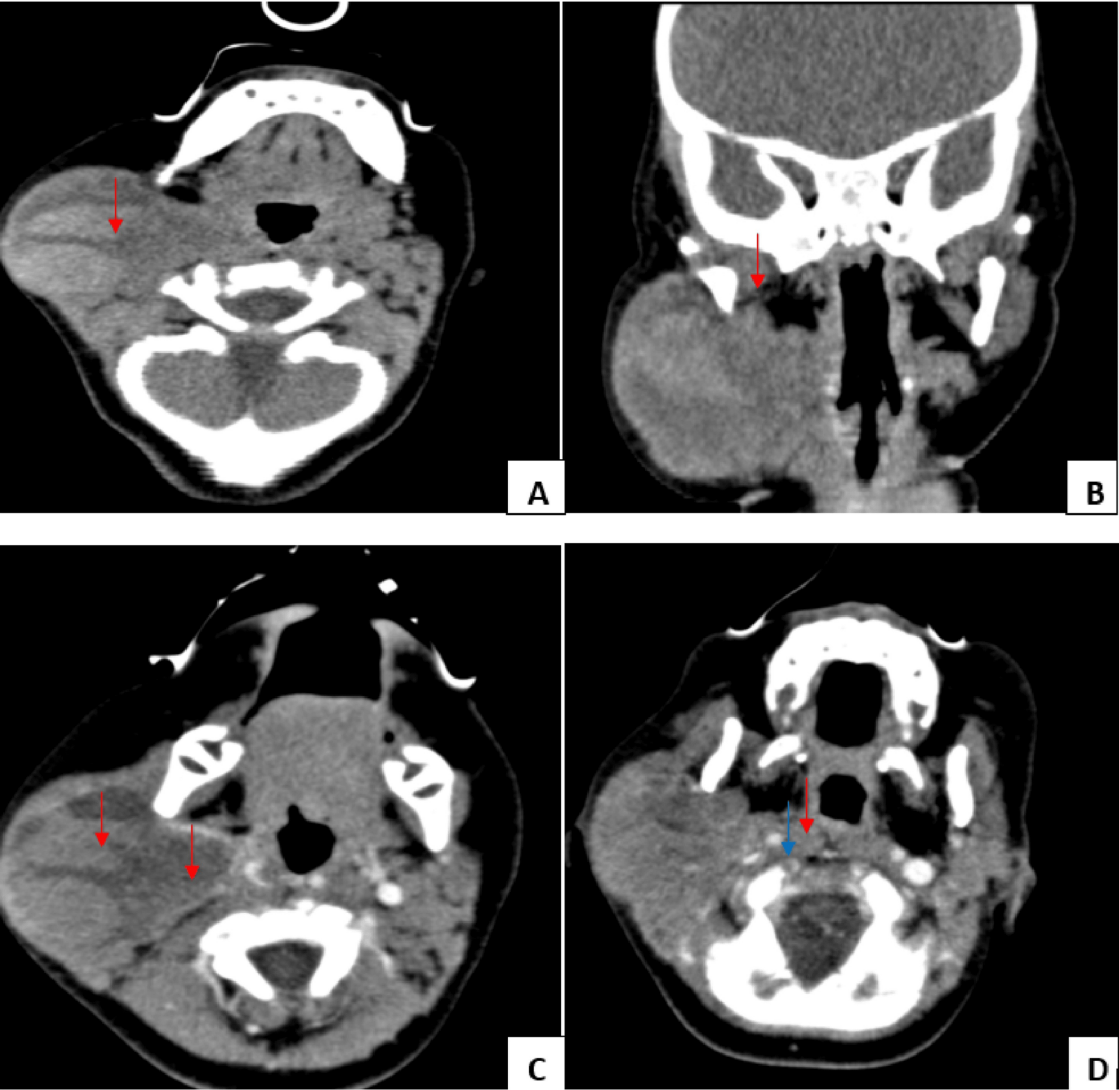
Facial CT scan A: Lesional process centered on the right parotid region (red arrow), well limited, of heterogenous density, with spontaneously hyperdense areas (hemorrhagic) delimiting foci of necrosis B: The process comes into contact with the temporomandibular joint (red arrow). C: After injection of the contrast agent (CA), this tumoral process discreetly enhances, delimiting foci of necrosis (red arrow). D: The lesional process encompasses the internal carotid artery, which remains of normal caliber and permeable (red arrow), and pushes back the internal jugular vein, which also remains permeable (blue arrow).
